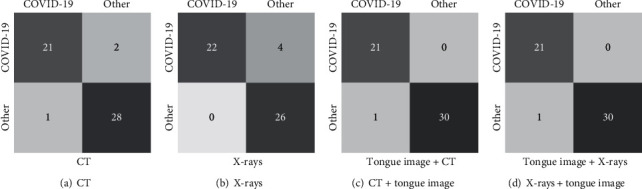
Confusion matrixes of CT-based and X-ray-based single-modality and multimodality classification.
